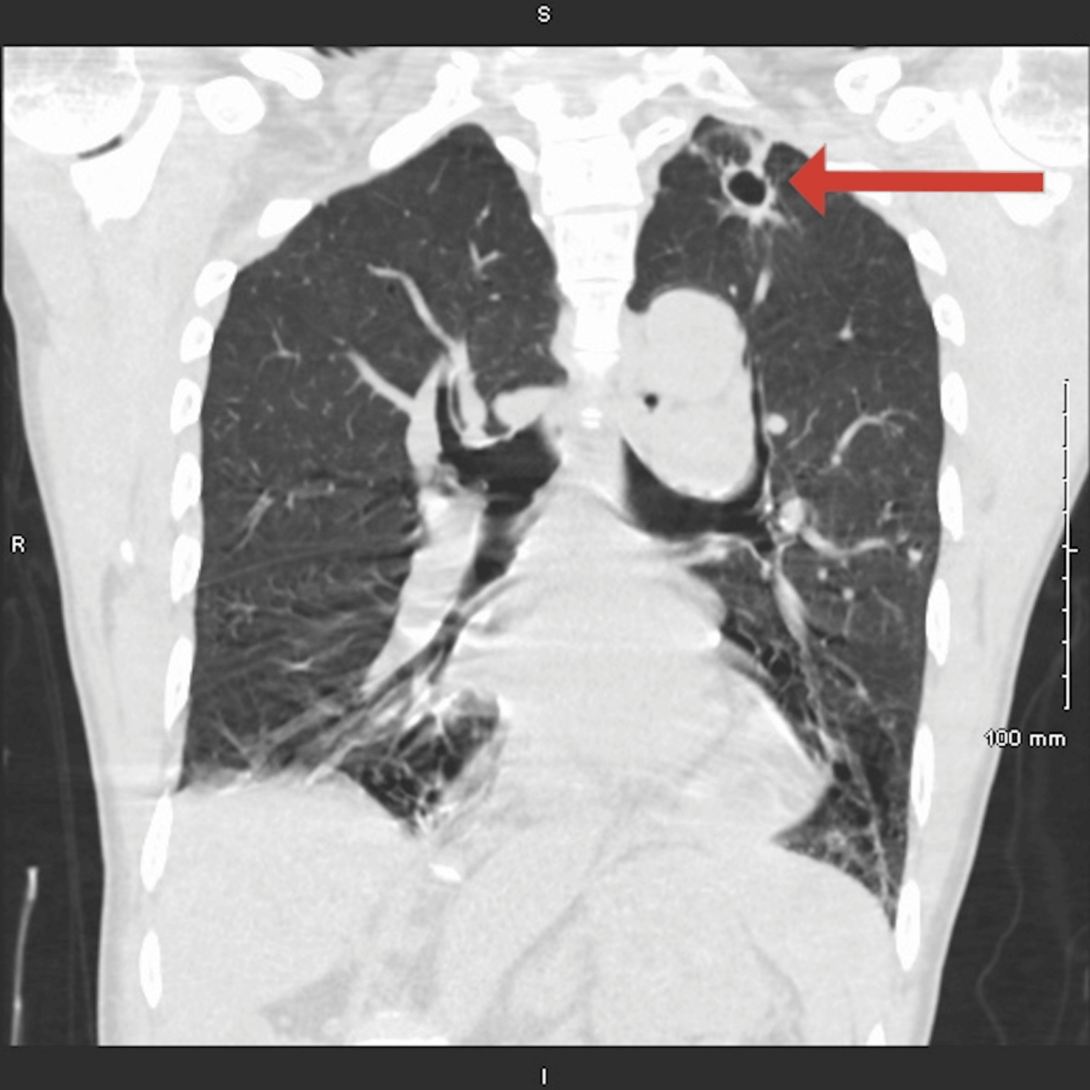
Non-contrast CT image of the chest demonstrating a cavitary lesion in the apex of the left lung, as indicated by the red arrow
